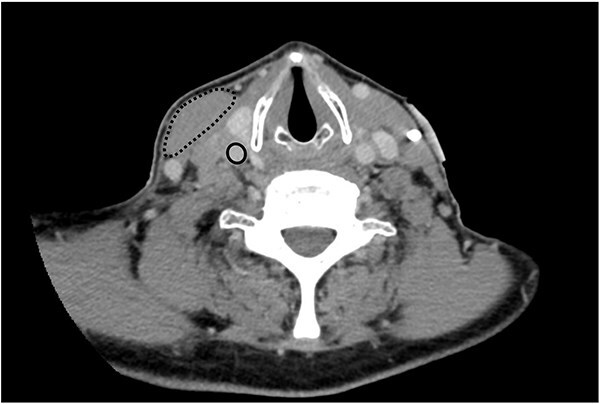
Axial plane image of contrast-enhanced CT scan demonstrating right-sided neck mass displacing the right sternocleidomastoid muscle (dashed line), occluding the right internal jugular vein (not seen) and compressing the right carotid artery (solid line) .
